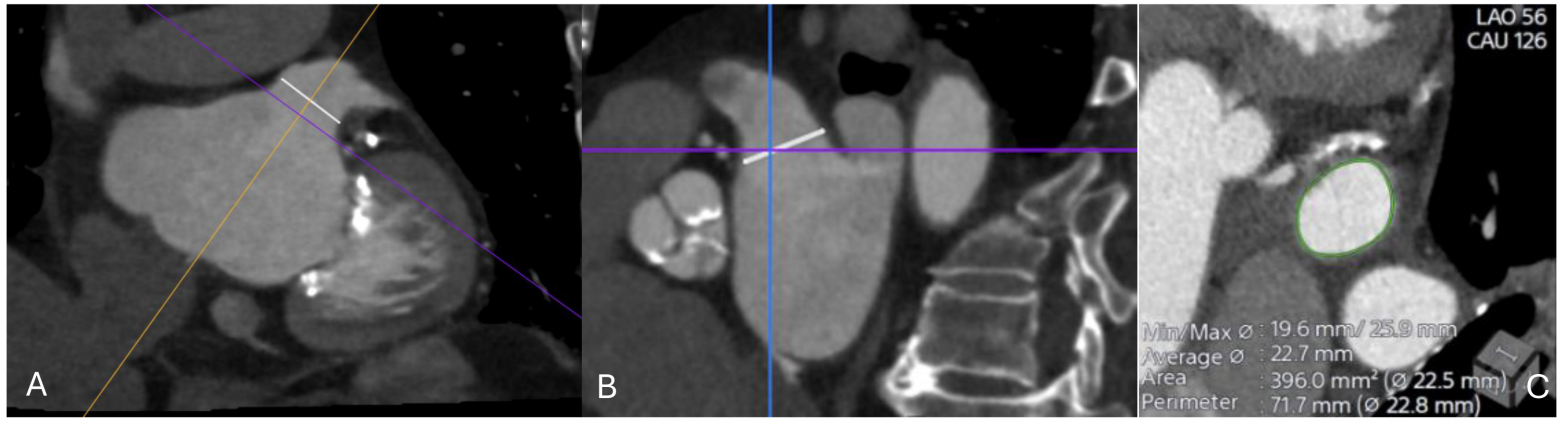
CT imaging assessment of the left atrial appendage (LAA) prior
